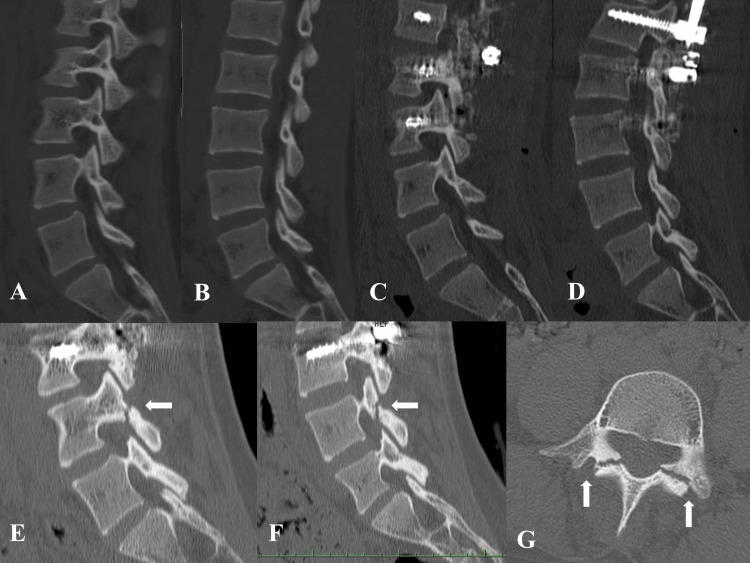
Case 1. Sagittal and axial CT images for the PI of L4. Preoperative and one week after surgery CT scans show no abnormalities in the pars interarticularis (PI) of L4 (A: preoperative right PI, B: preoperative left PI, C: right PI one week after surgery, and D: left PI one week after surgery). CT at the onset of low back pain, 43 months postoperatively, shows bilateral spondylolysis of L4 (E: right PI, F: left PI, and G: L4 axial). PI: pars interarticularis
